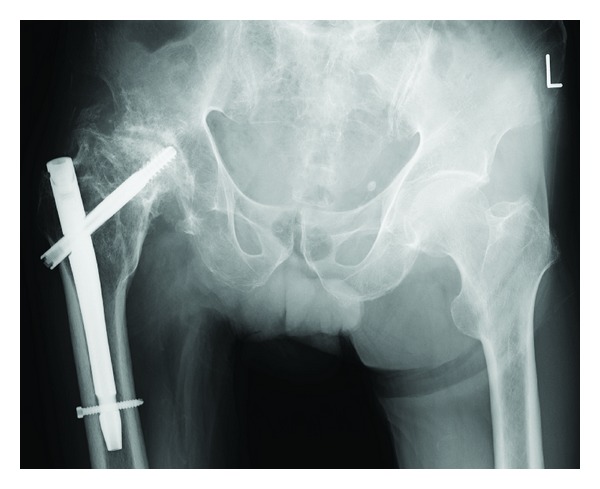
Anteroposterior radiograph captured 8 months after the operation showing strong bone union.
